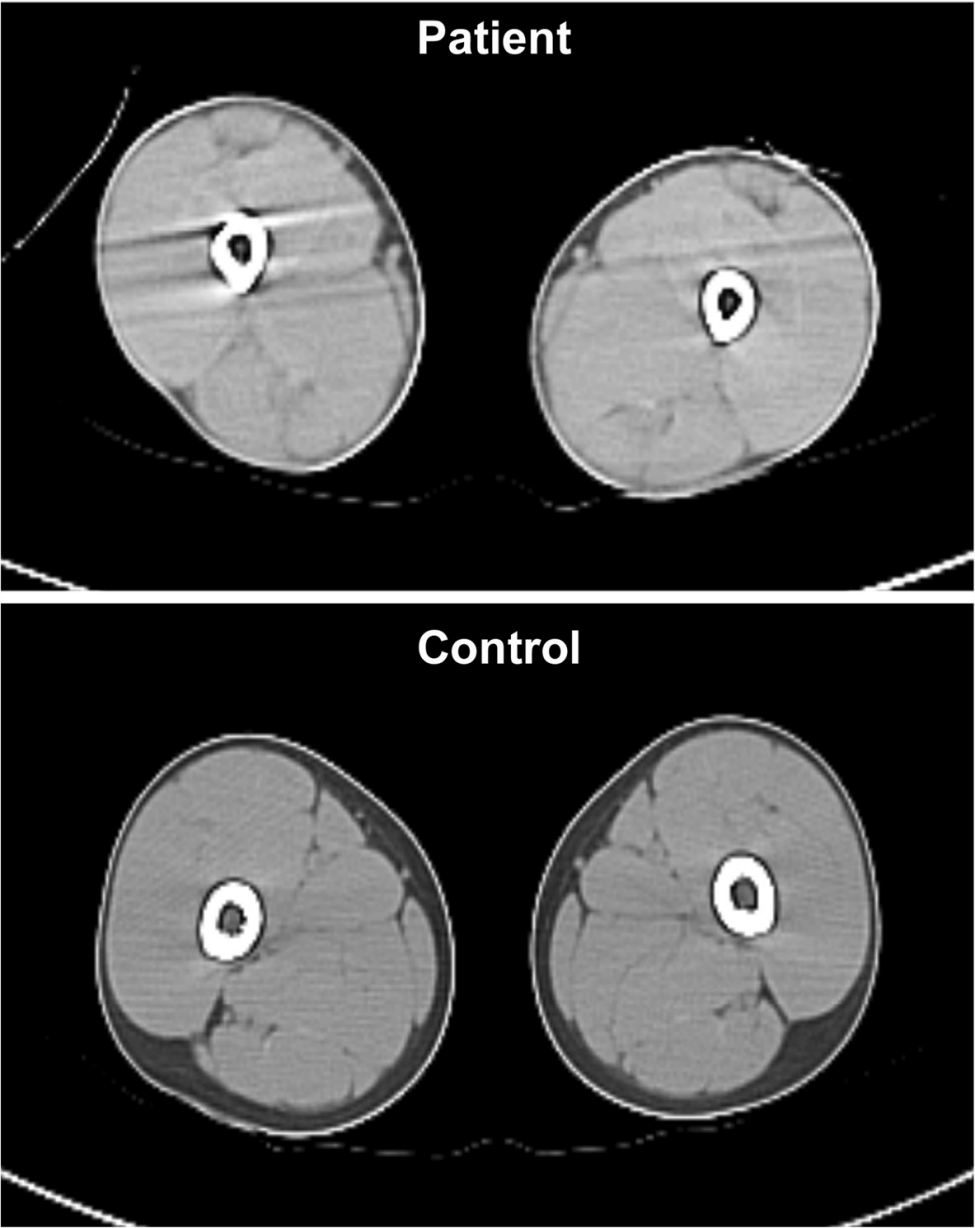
Lower limb CT, showing muscle hypertrophy and marked loss of subcutaneous fat.
